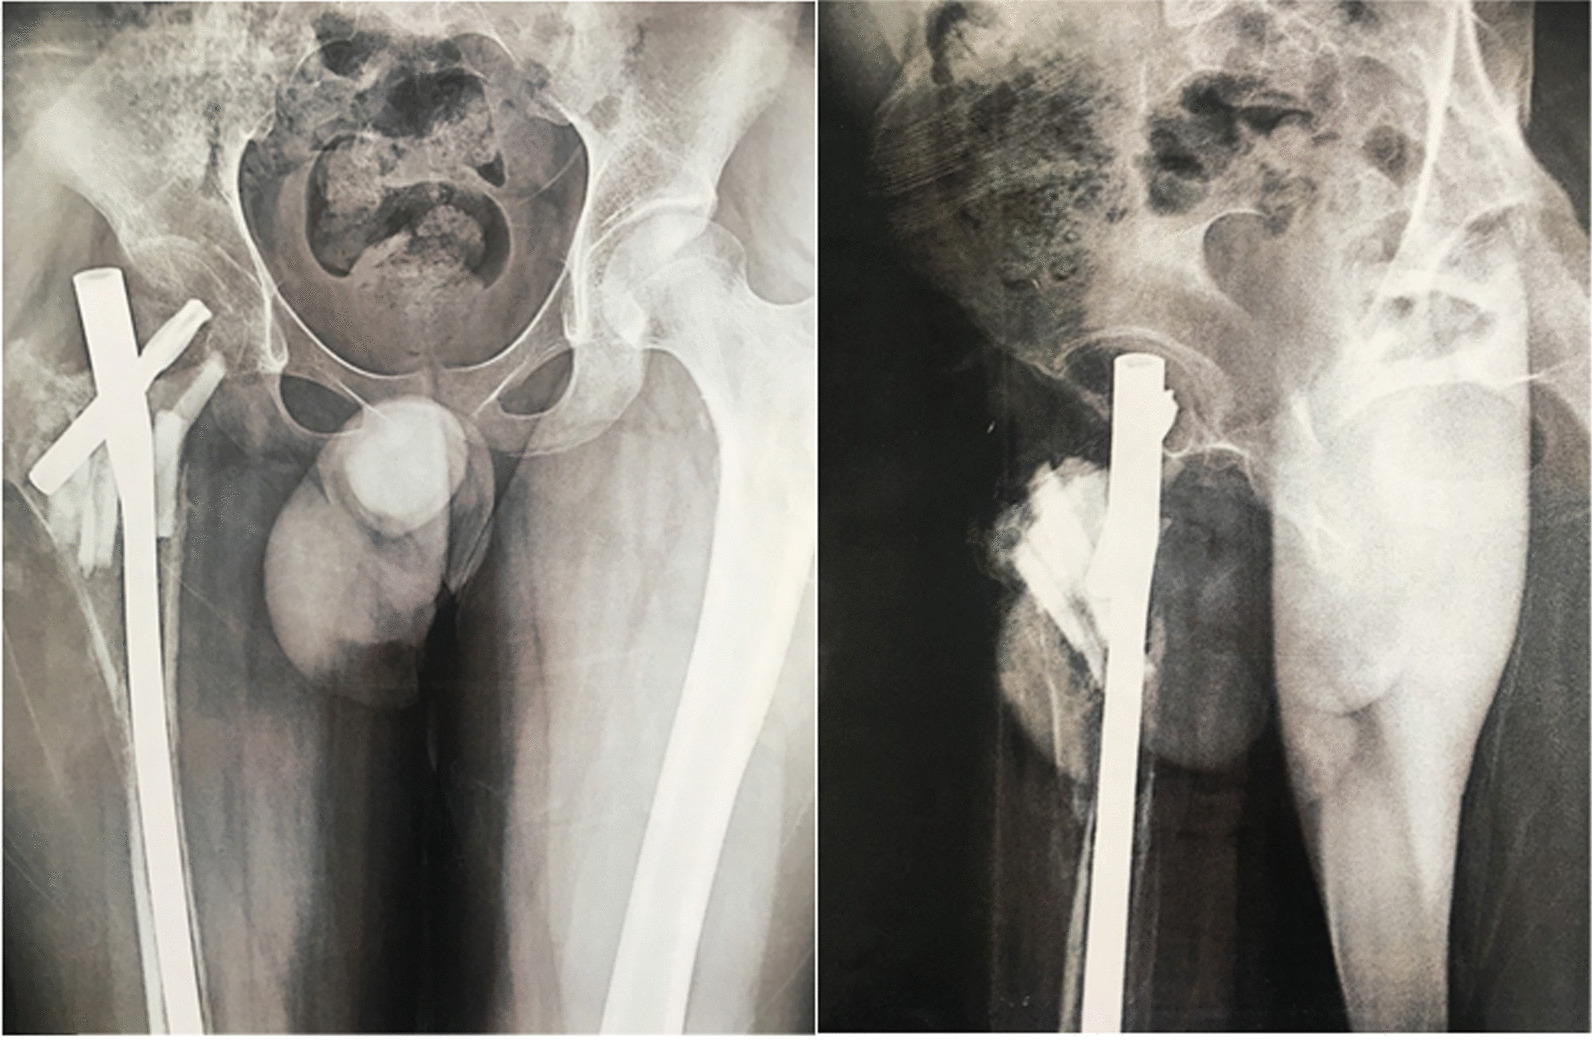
Radiographs of the right hip after revision surgery; note proper reconstruction of the intertrochanteric and femoral neck region by utilizing both structural allograft and cephalomedullary nail
